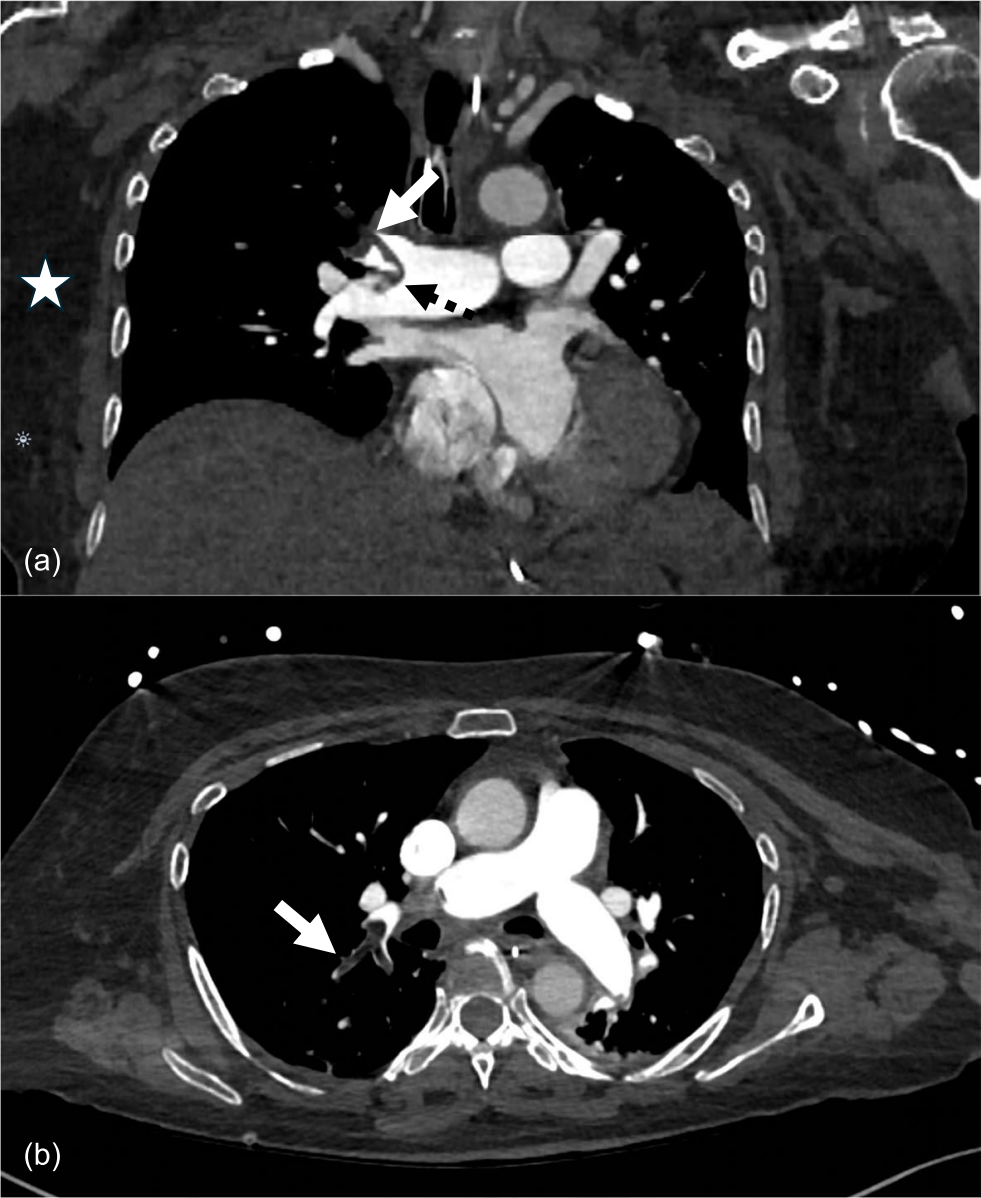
CT pulmonary angiogram. a Coronal soft tissue window demonstrating straddling pulmonary embolism within the right lobar and proximal segmental upper lobe branch (black dotted arrow). The filling defect in the right upper lobe lobar artery (white arrow) is of similar density to chest wall subcutaneous fat (white star). b Axial soft tissue window demonstrating the heterogeneous appearance of the embolus, suggesting admixed bland thrombus (white arrow)
